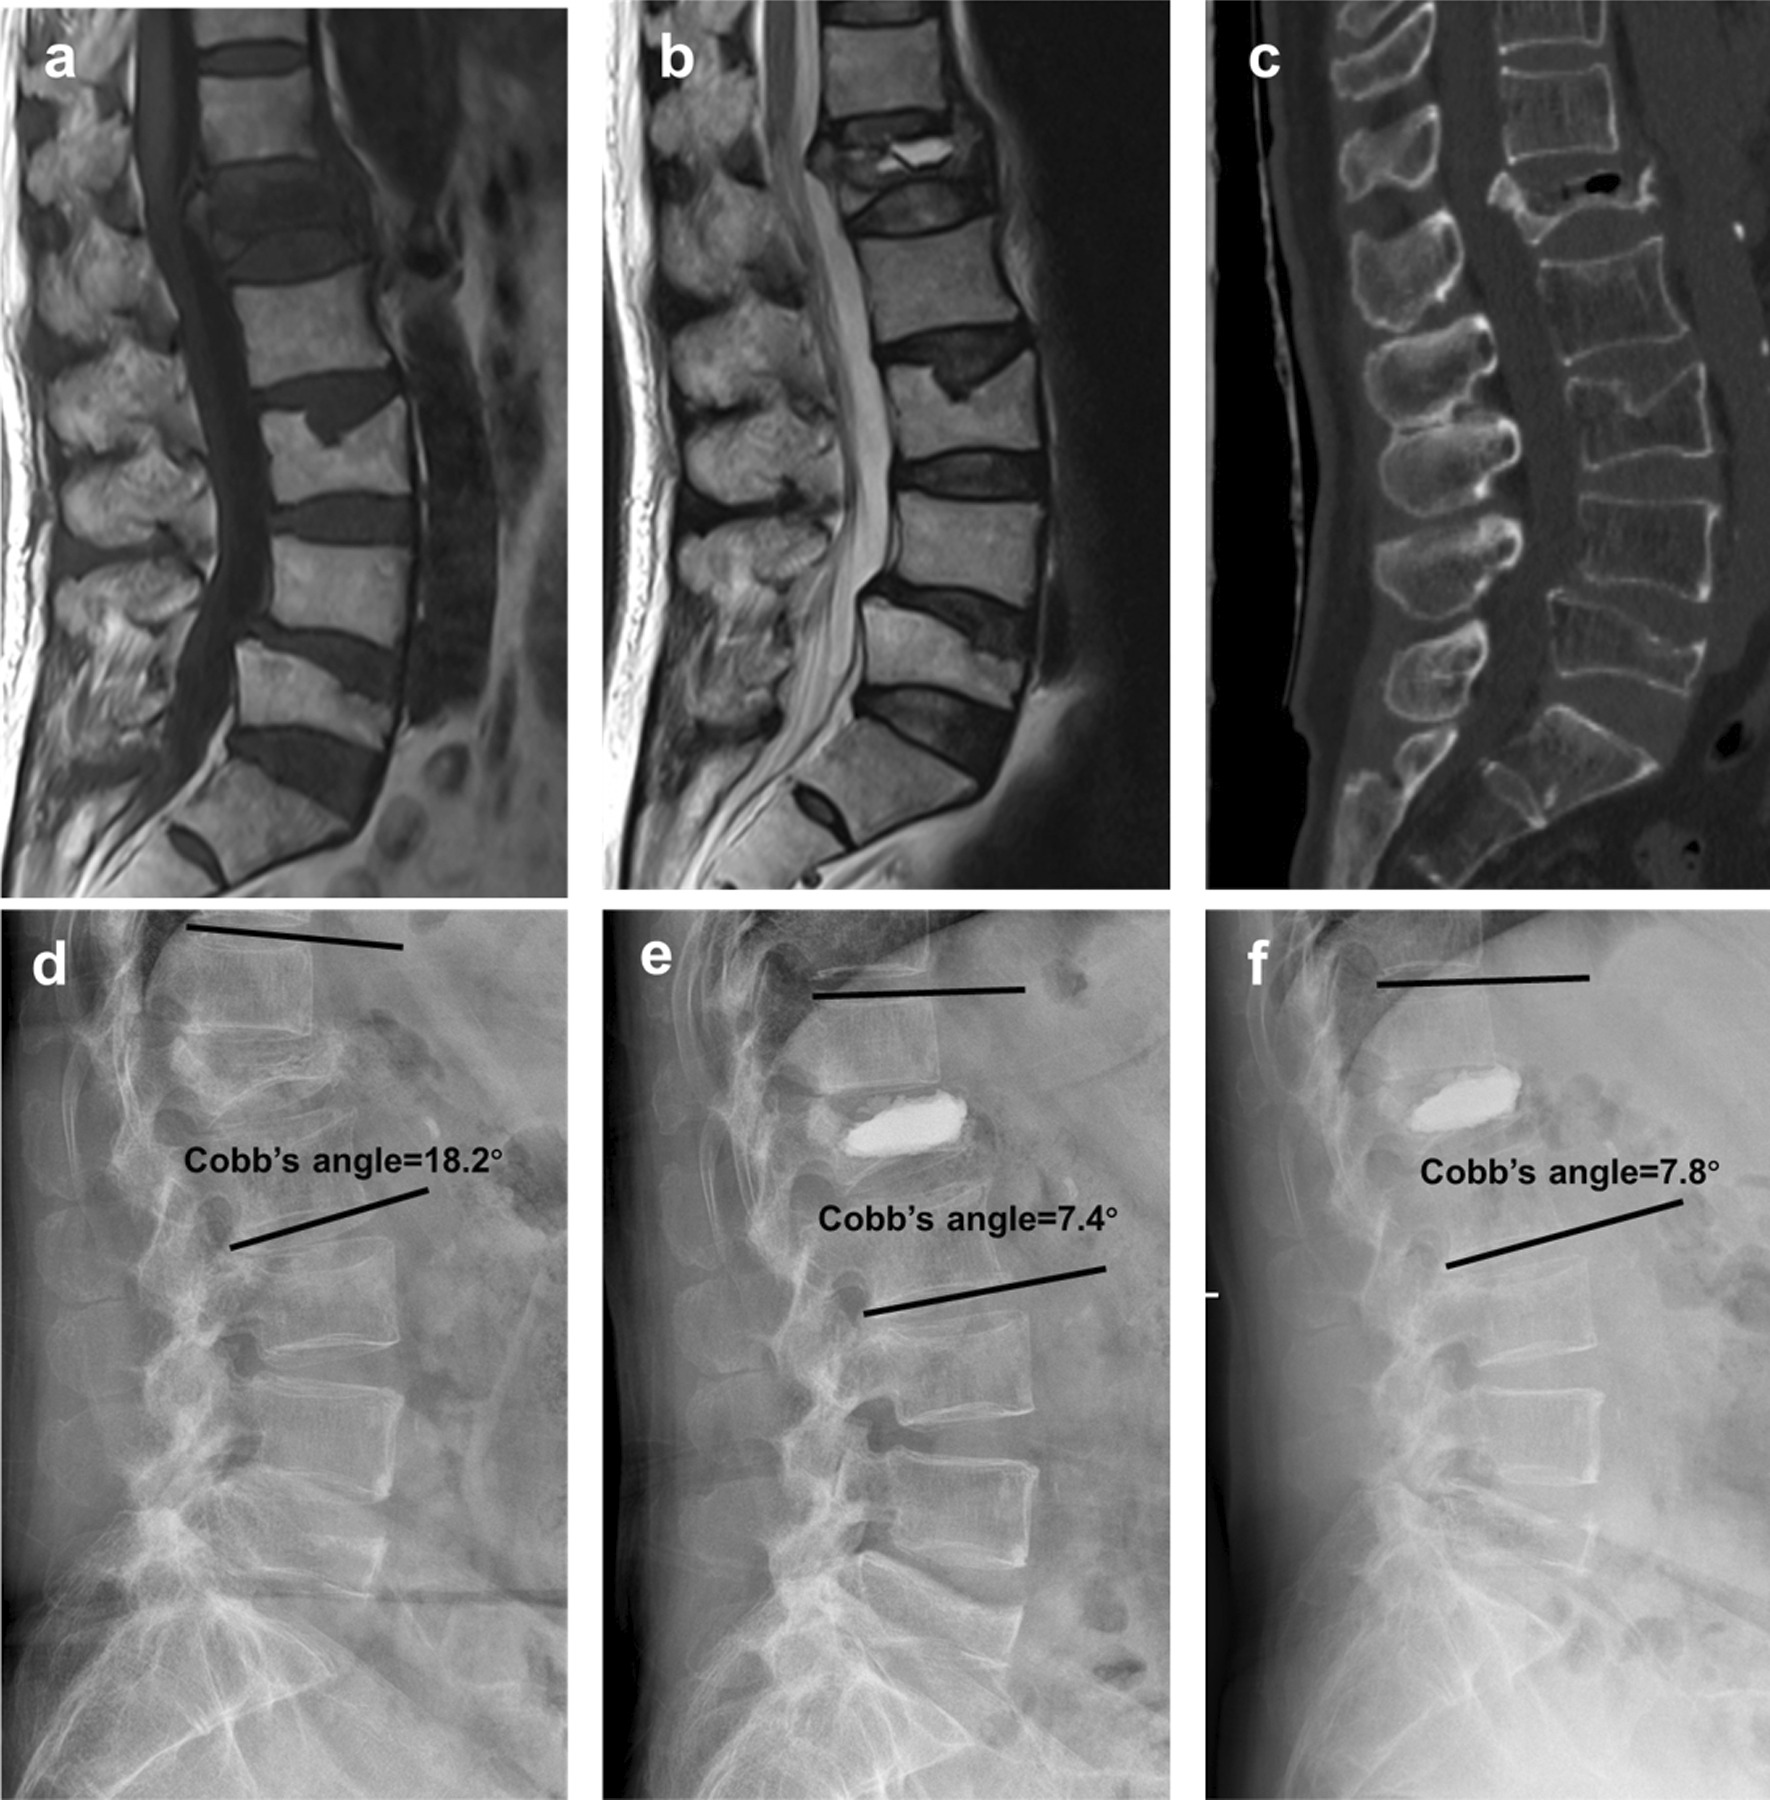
A 64-year-old woman who had L1 stage III Kummell disease without neurological symptom was treated with PKP. a–c The preoperative MRI T1WI, MRI T2WI and CT films showed a chronic osteoporotic vertebral compressive fracture. d–f The preoperative, postoperative and final follow-up X-ray films displayed the vertebral height and Cobb’s angle was well recovered
